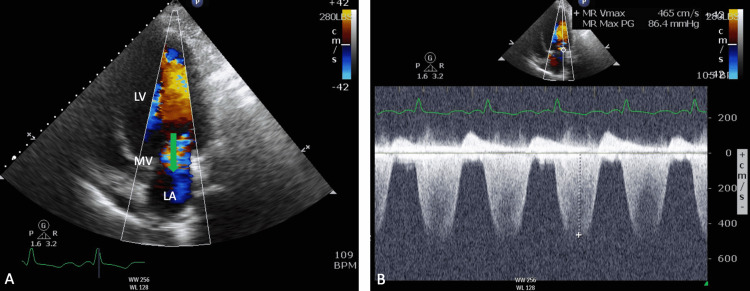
Apical two-chamber view from TTE showing severe mitral regurgitation jet (green arrow) from LV to LA. (B) Continuous wave Doppler of the regurgitant jet with low velocity and a triangular profile, suggestive of severe MR. TTE, transthoracic echocardiogram; LA, left ventricle; LV, left ventricle; MV, mitral valve; MR, mitral regurgitation
